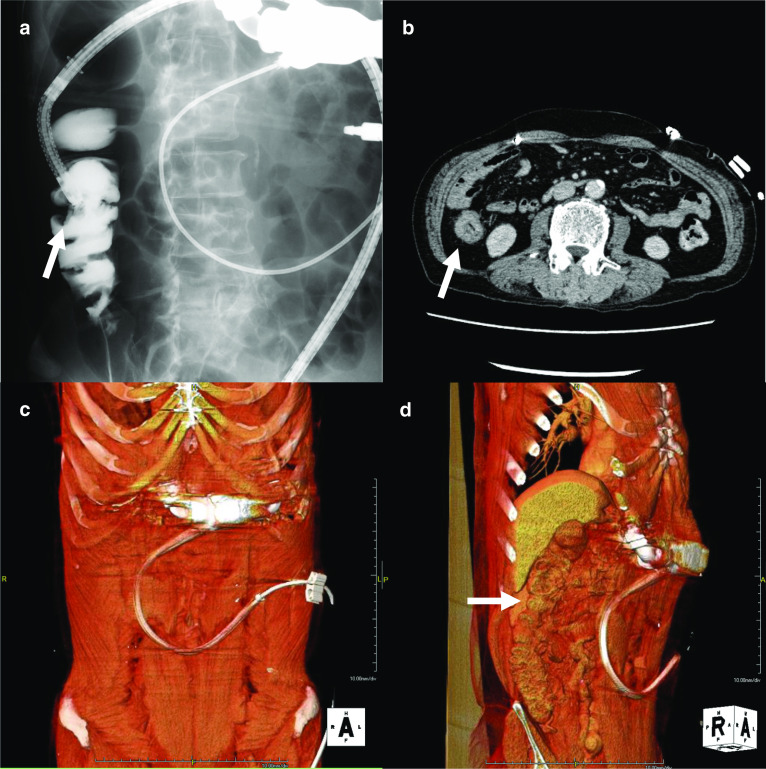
The positional relationship between the tumor and the driveline by X-ray (a) and CT images (b). The tumor (arrow) is away from driveline. c, d The positional relationship between the tumor (arrow) and the driveline by 3D CT images
